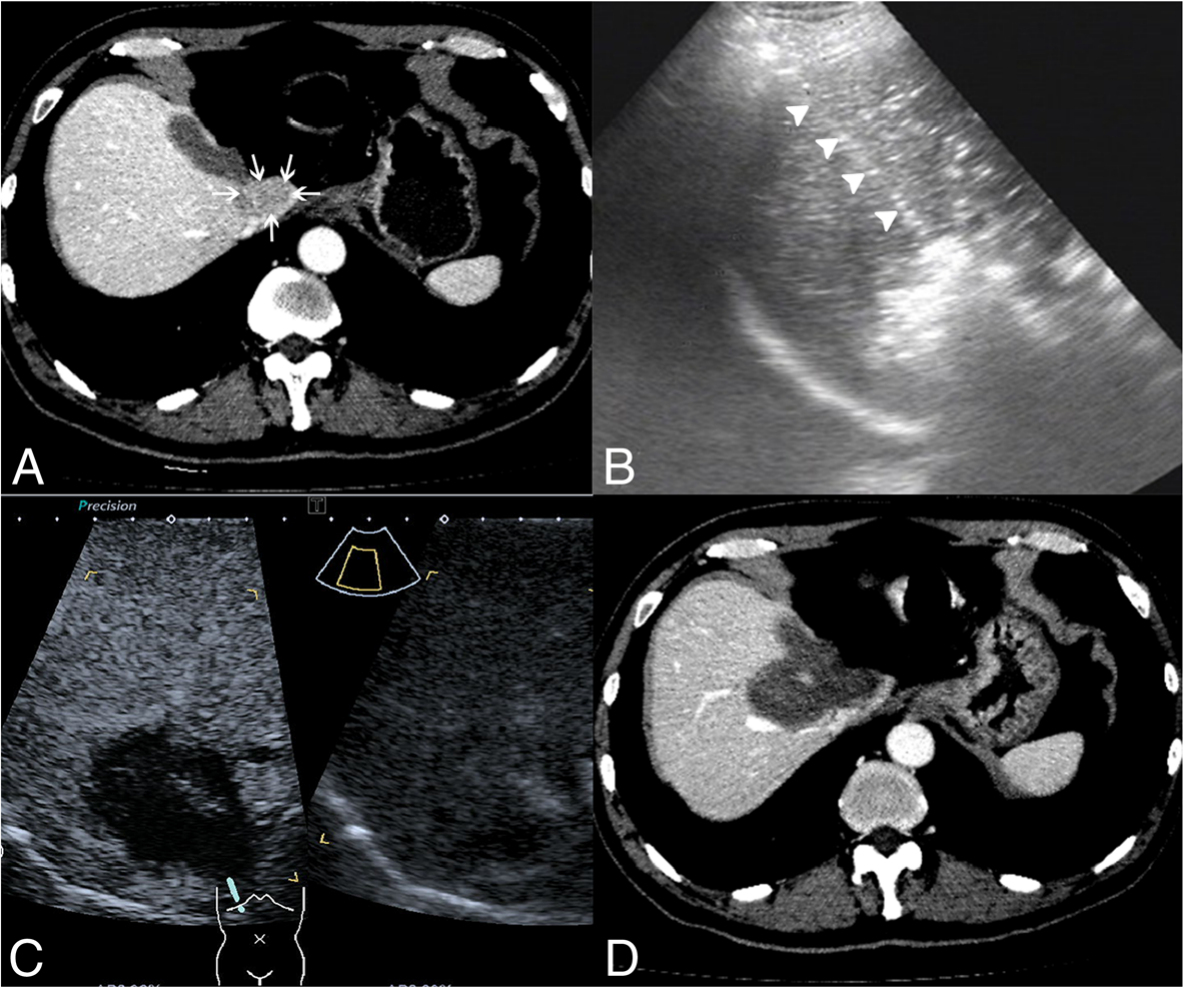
Images of a patient with recurrent hepatocellular carcinoma (HCC) in the caudate lobe treated by percutaneous radiofrequency ablation (RFA). a transverse contrast-enhanced computer tomography (CT) before treatment shows the tumor in the caudate lobe (arrow); b tumor appears completely hyperechoic after the beginning of RFA, and the electrode (arrowhead) is clearly showed on the ultrasound image; c CEUS image obtained 1 month after RFA shows the tumor completely ablated; d contrast-enhanced CT image obtained 1 month after RFA shows the tumor completely ablated
